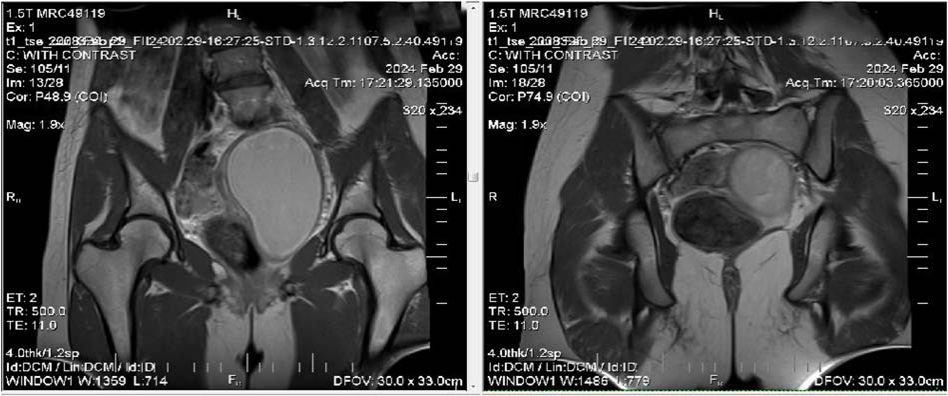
MRI imaging showing a big pelvic cystic hematoma measuring (7 cm × 12 cm × 6.7 cm) in the left paracentral position that extends close to the vaginal opening to the level of the right adnexa, encircled by a regular, thick wall that did not luminesce, and a relatively small myometrium with a regular endometrium.
